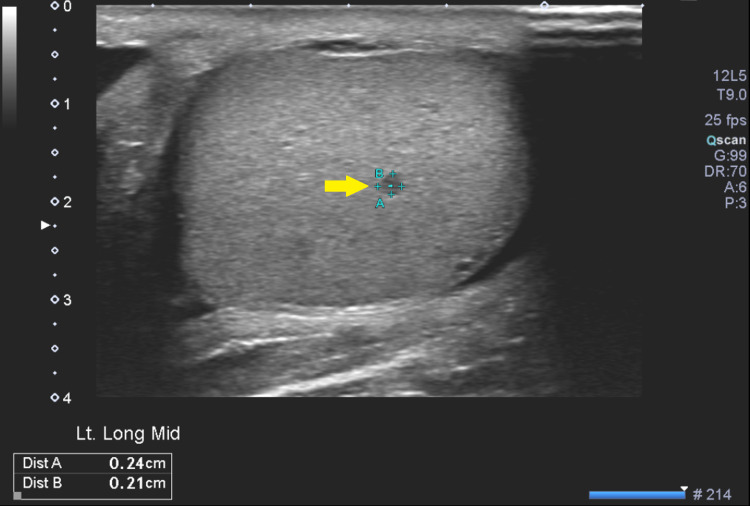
Testicular ultrasound showing typical hypoechoic lesion (with yellow arrow) in the patient.
